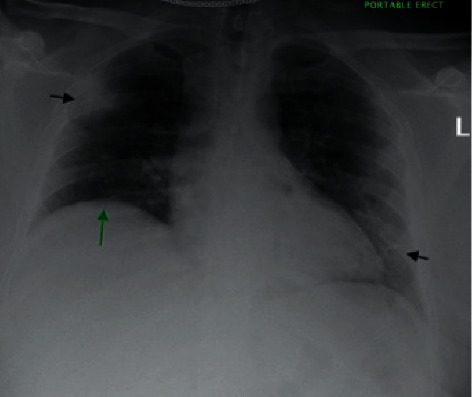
A portable chest radiograph on day 1 of presentation shows bilateral patchy infiltrates (black arrows) in the right upper lung and left lower lung, with mild elevation of the right hemidiaphragm (green arrows).
